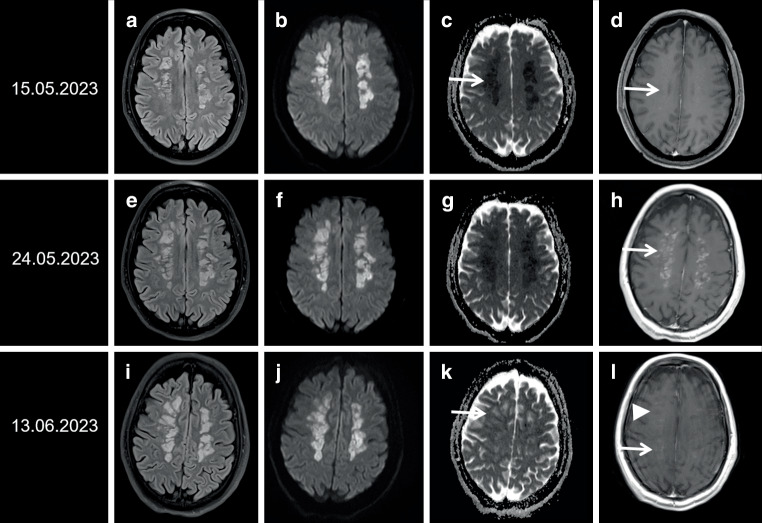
Sequential MR imaging in a 29-year-old woman suffering from severe toxic leukoencephalopathy. a, e, i Axial fluid attenuated inversion recovery (FLAIR); b, f, j and c, g, k axial diffusion weighted images (DWI; b = 1000 s/mm^2^) and corresponding apparent diffusion coefficient (ADC) maps; d, h, l axial T1 weighted images (WI) after administration of contrast agent. a–d Initial MR imaging 3 days after drug intake showing partially conflating bilateral hyperintense signal changes in the centrum semiovale (a) with severe restricted diffusion (b, c; ADC mean: 0.23 × 10^−3^ mm^2^/s, *arrow*; normal: 0.72 × 10^−3^ mm^2^/s); slight punctuate contrast enhancement (d, *arrow*). e–h Follow-up imaging on day 12 disclosed progressive hyperintense lesions (e, f), slight improvement of lowered ADC values (g; ADC mean: 0.37 × 10^−3^ mm^2^/s) and distinct patchy contrast enhancement (h, *arrow*). i–l On day 32 FLAIR images exhibit explicit bilateral hyperintense signal changes (i) with “shine through effect” on DWI (j), pseudonormalization of ADC values (k, *arrow*; range: 0.79–1.44 × 10^−3^ mm^2^/s) and decreasing contrast enhancement (l, *arrow*); note inhomogeneous lowering of T1 signal in the centrum semi-ovale (l, *arrowhead*)
